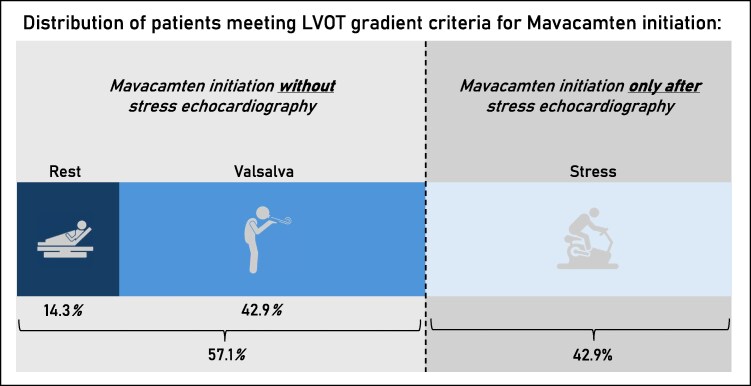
Distribution of echocardiographic conditions prompting mavacamten initiation. Proportion of patients meeting treatment-qualifying left ventricular outflow tract obstruction (LVOTO) criteria based on resting echocardiography, Valsalva-provocation, or exercise echocardiography. In 57.1% of patients, treatment eligibility was established without the need for exercise echocardiography (rest: 14.3%; Valsalva: 42.9%), whereas in 42.9% of patients, a qualifying LVOT gradient was documented exclusively during exercise echocardiography
